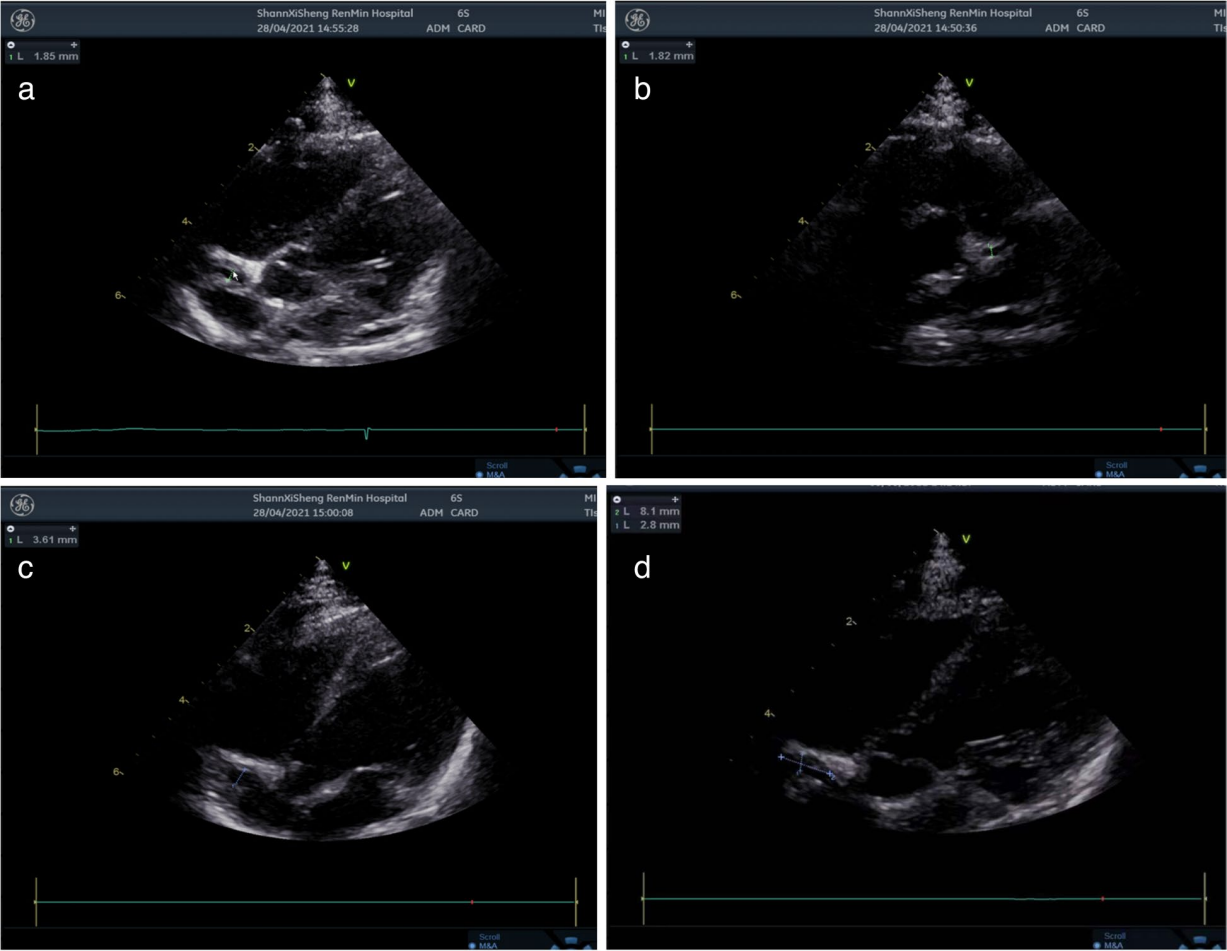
Echocardiography on day 4 of course of disease. a Apical five chamber view showing dimensions of the right coronary artery. b Parasternal short axis view showing left anterior descending branch. c Apical five chamber view showing dimensions of the proximal right coronary artery. d Apical five chamber view showing the intramural throbosis
